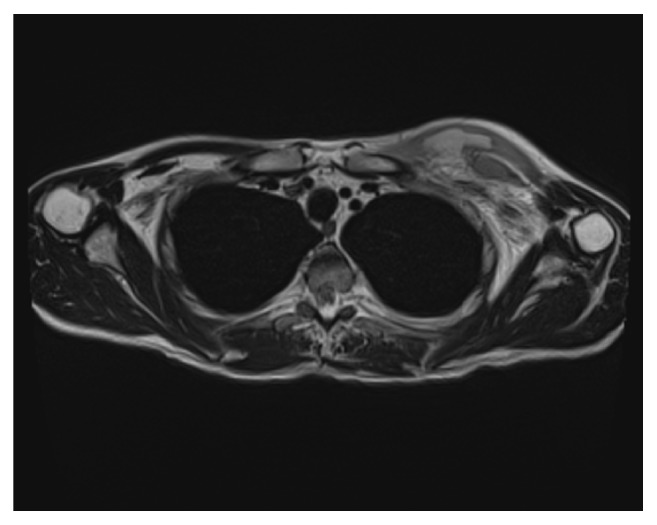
Magnetic resonance image.
